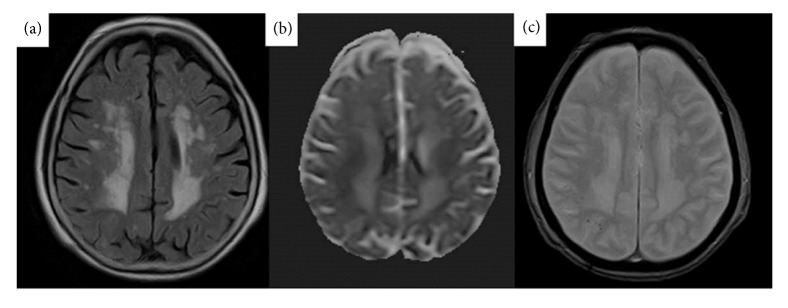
A follow-up brain magnetic resonance imaging (MRI) performed after 4 months shows a reduction of hyperintensity on fluid-attenuated inversion recovery (FLAIR; (a)) and apparent diffusion coefficient (ADC; (b)) in the right parietal lobe. The reduced subcortical microbleeds in the right parietal lobe are noted (c).
